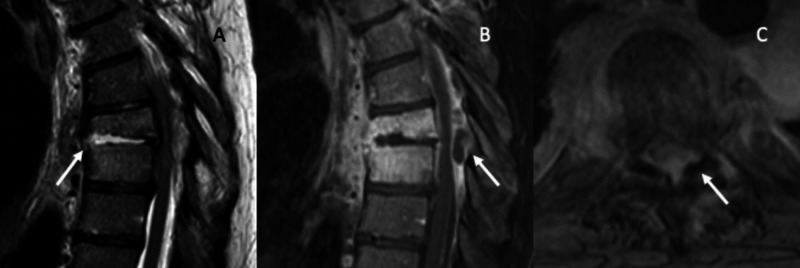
Initial imaging Preoperative imaging at initial presentation. A: sagittal T2 MRI showing discitis/osteomyelitis at T8/9 (arrow showing vertebral collapse). B: sagittal T1 with contrast MRI showing osteomyelitis/discitis at T8-9, arrow showing dorsal epidural abscess with severe spinal cord compression. C: axial T1 with contrast MRI demonstrating the severity of cord compression, arrow redemonstrating dorsal epidural abscess.
